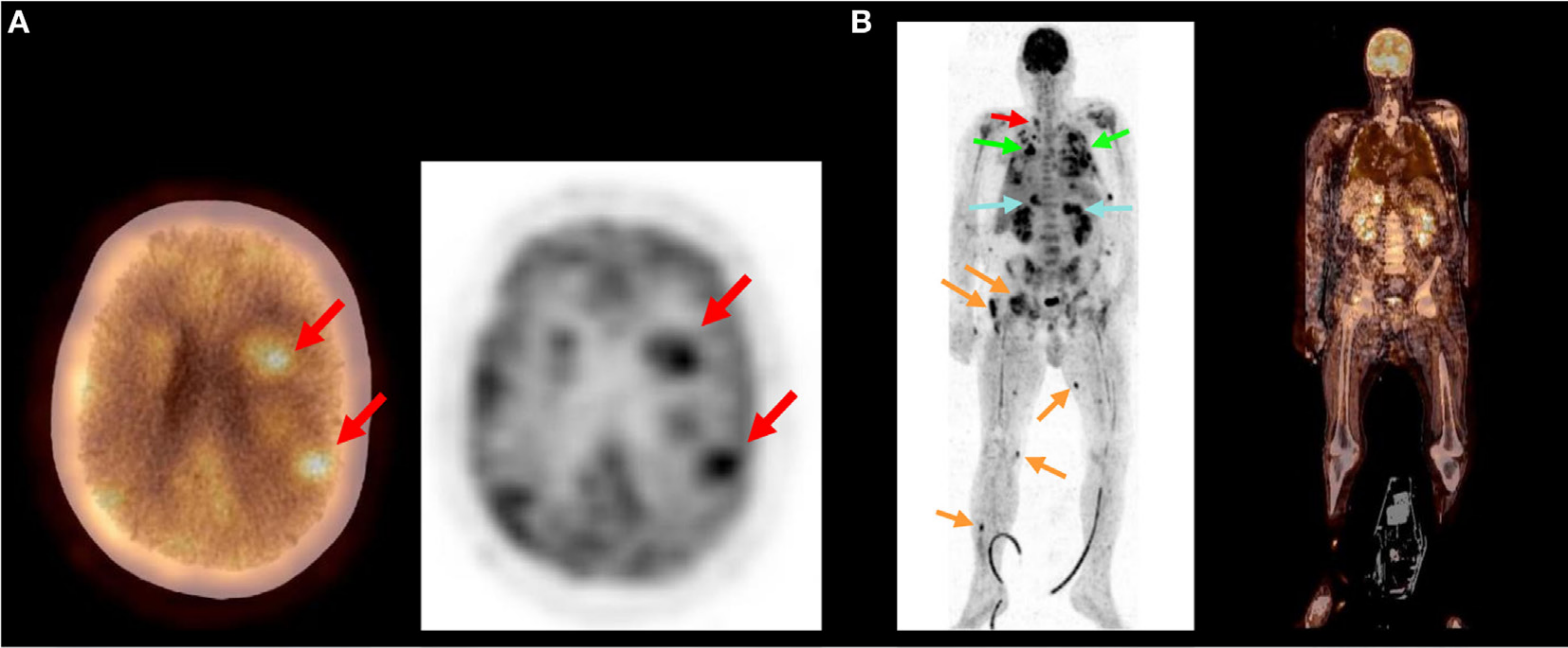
This 57-year-old man presented with fever and shortness of breath. Antibiotic treatment was initiated. Shortly, he developed right sided hemiparesis and confusion. Cultures remained sterile. (A) PET/CT revealed multiple cerebral and cerebellar abscesses. (B) Further abscesses were identified throughout the body: at the side of the thyroid gland (red arrow), within the lung (green arrows), liver, and both adrenal glands (blue arrows) as well as multiple foci within muscles (orange arrows). A nocardiosis was suspected. *Nocardia farcinia* was eventually cultured from a bronchoalveolar lavage confirming the diagnosis.
